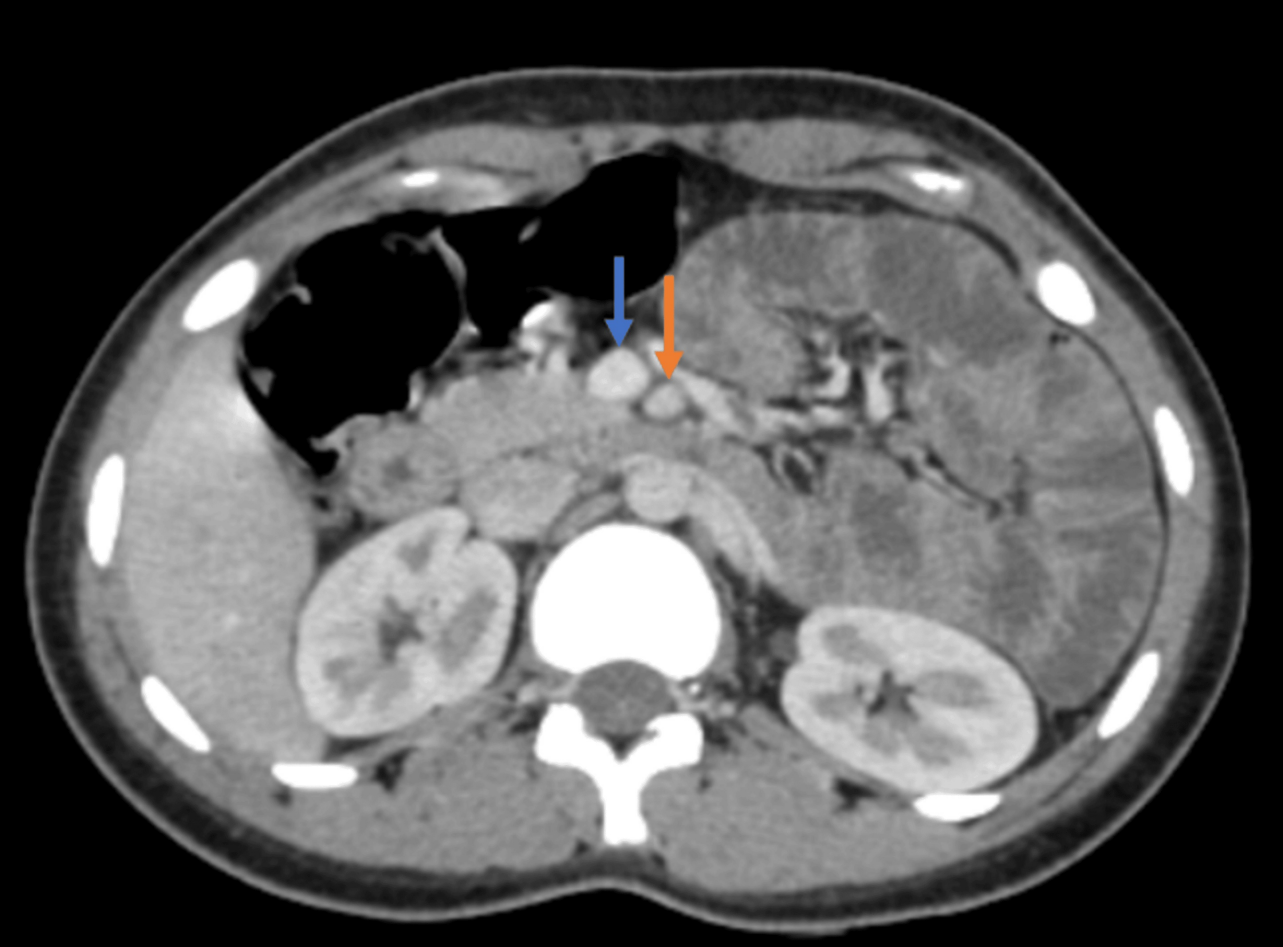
Contrast-enhanced axial CT abdomen (porto-venous phase) Contrast-enhanced axial CT abdomen image demonstrating a preserved superior mesenteric artery (orange arrow) and superior mesenteric vein (blue arrow) relationship.
